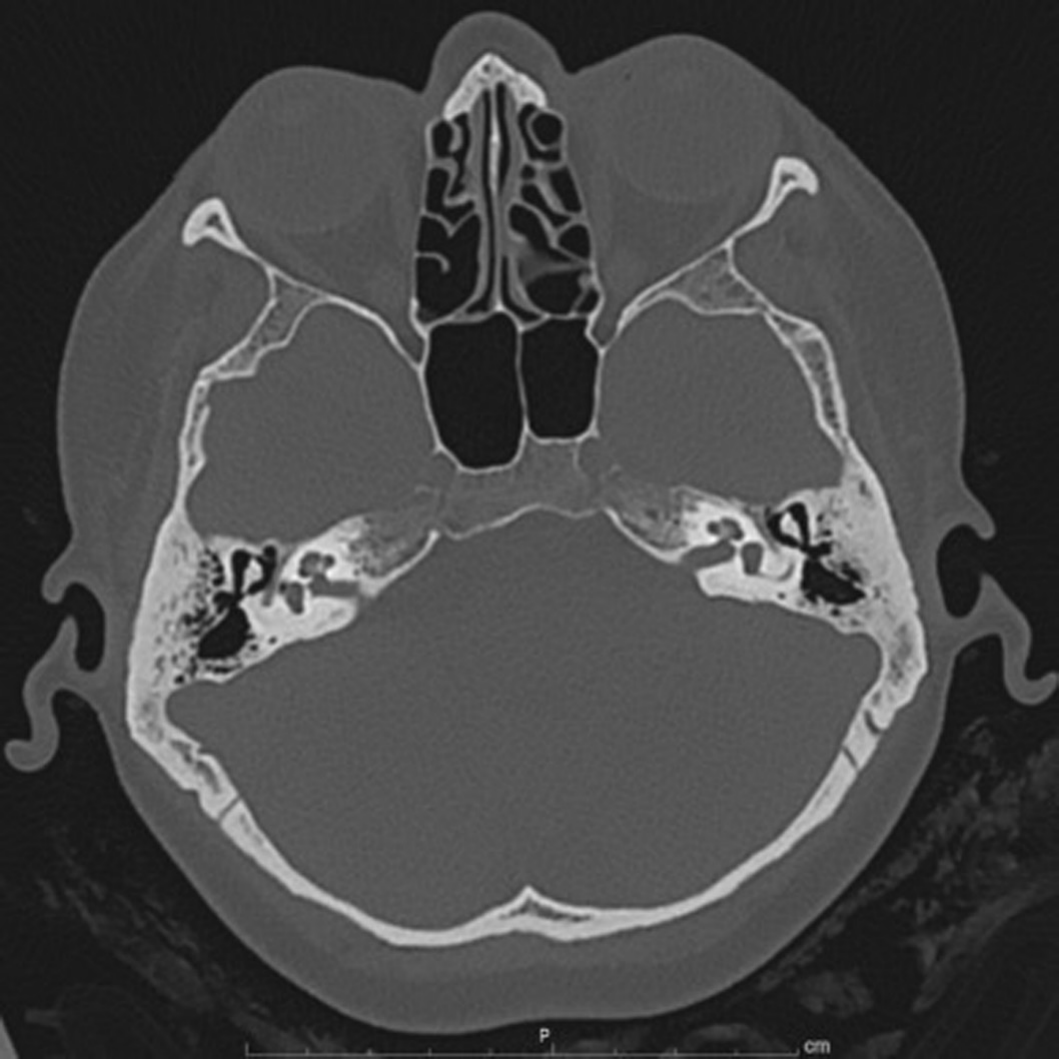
Ear high‐resolution CT scan with no evidence of dehiscence of the tympanic and mastoid tract of the right facial nerve.
